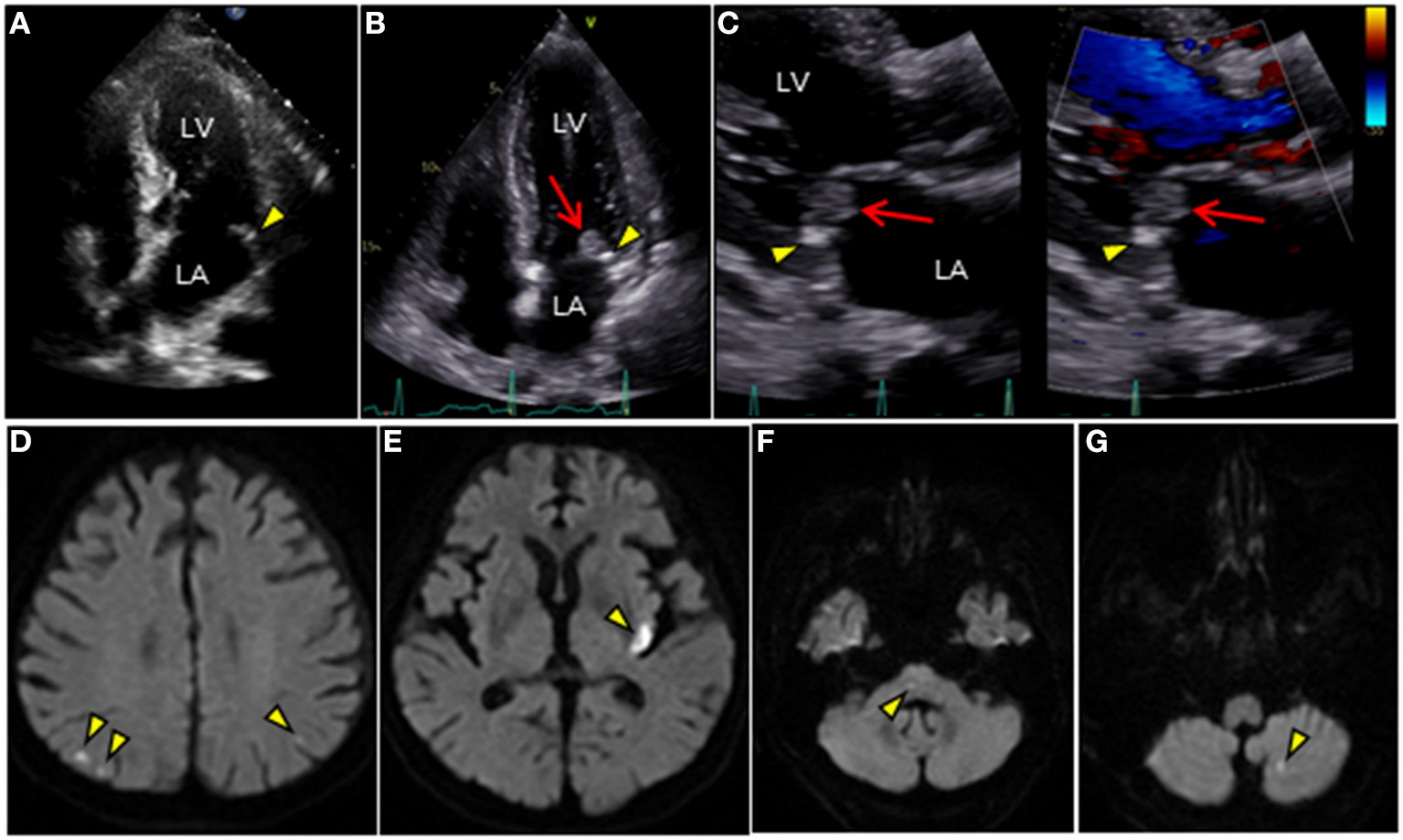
TTE and brain MRI. (A–C) Longitudinal TTE: (A,B) apical 4-chamber and (C) parasternal long-axis views. (A) TTE shows tiny MAC along the posterior mitral annulus 1 year ago (arrowhead). (B,C) TTE after admission shows MAC progression (arrowhead) and a large round mass superimposed on the MAC (arrow). Mass with wide attachment (10 ×11 mm in size) is characterized by heterogeneity and oscillation. (C) Notably, color Doppler TTE showed no significant mitral regurgitation. (D–G) Brain diffusion-weighted MRI detects multiple acute cerebral infarctions in both hemispheres (arrowheads). LA, left atrium; LV, left ventricle; MAC, mitral annular calcification; MRI, magnetic resonance imaging; TTE, transthoracic echocardiography.
